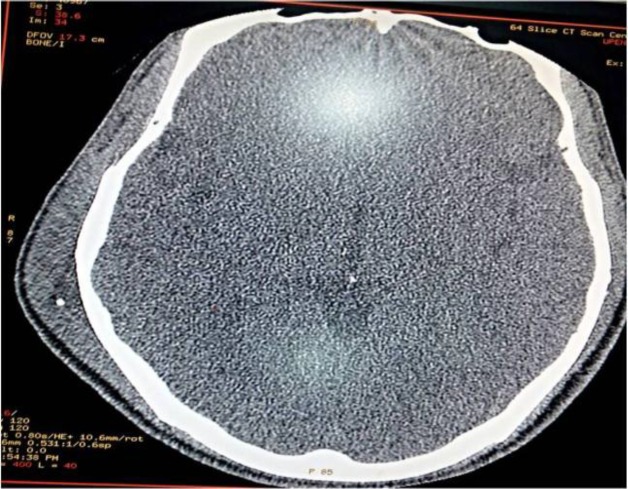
CT scan of the temporal bone showing unifocal calcification on the right side within the substance of the temporalis muscle with swelling.
